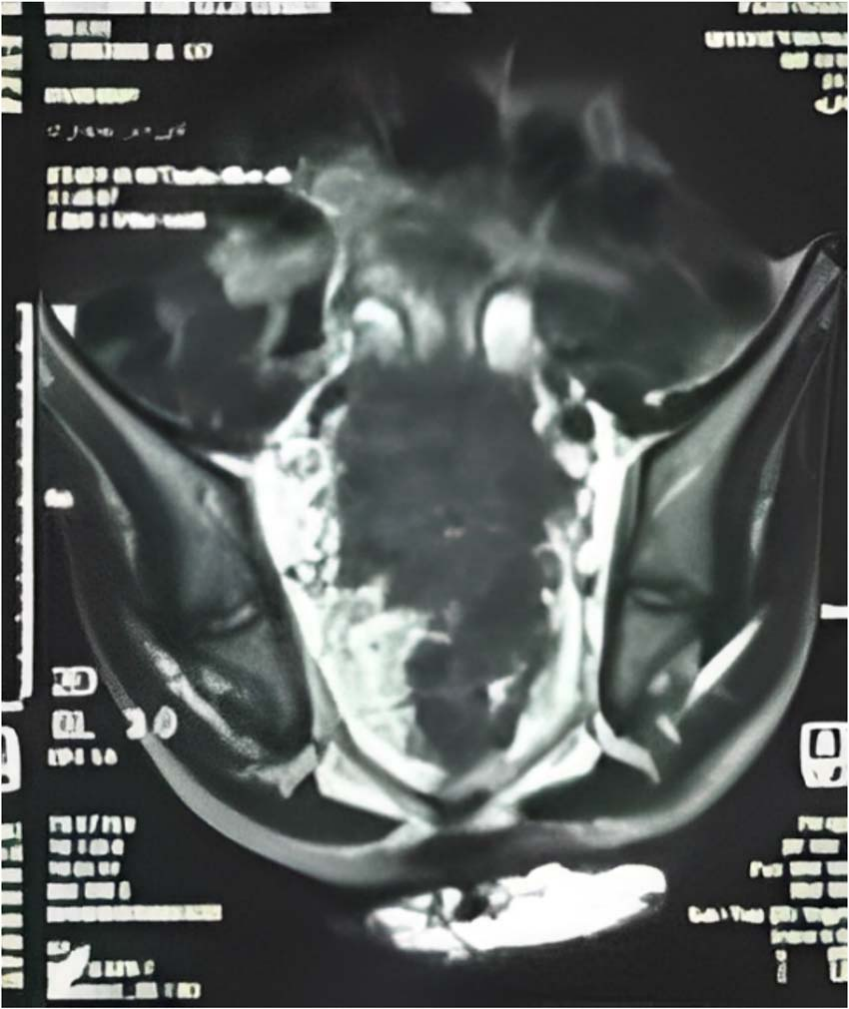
MRI sagittal view showing the tumor extending from the rectosigmoid to the upper and middle rectum, with mesorectal lymph nodes and extramural venous invasion.
